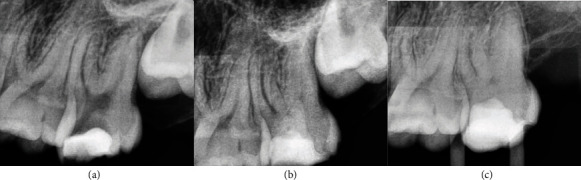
Permanent tooth 27. The image (a) shows diagnostic X-ray, image (b) immediate postoperative, and image (c) control at 39 months.
